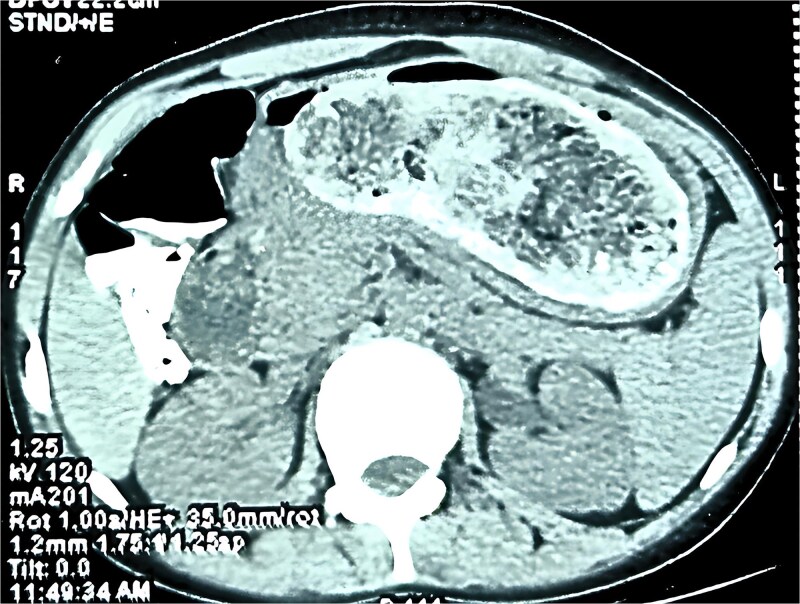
CT scan showing the bezoar within the stomach/intestine.
